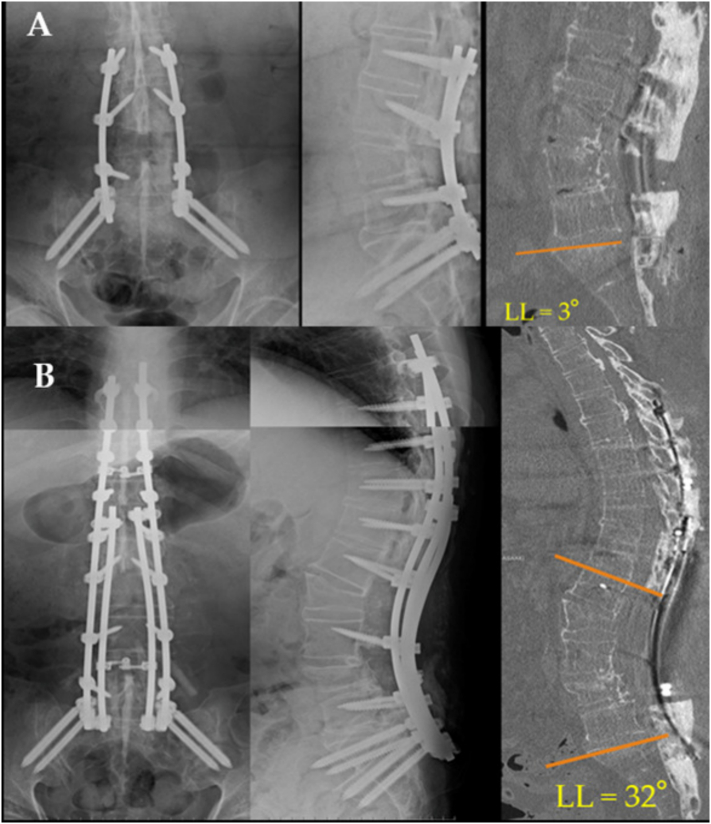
A) Frontal and lateral radiographs after the 1st stage operation showing increased lumbar lordosis to 3° by L4 PSO and posterior instrumentation from L2 to the pelvis. B) Frontal and lateral radiographs after the 2nd stage operation showing increased lumbar lordosis to 32° by L2 PSO and posterior instrumentation from Th8 to the pelvis.
